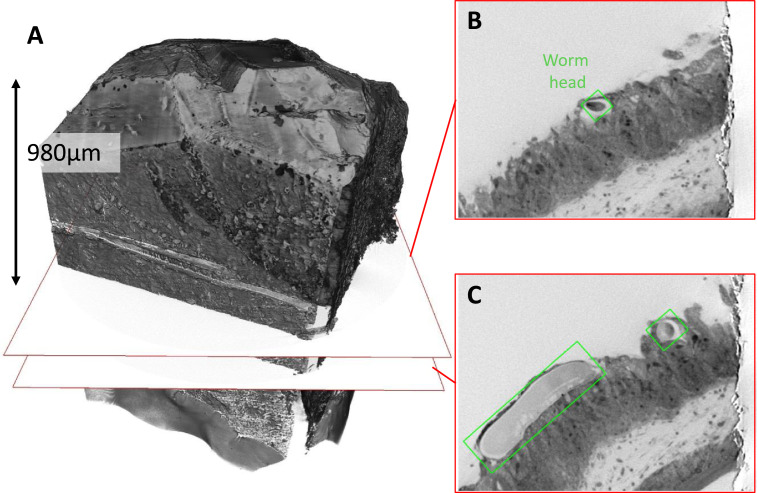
Identifying regions of interest within the X-ray data set to target subsequent trimming.*(A) A magnified volume render of the resin sample generated from the X-ray data. (B) Virtual XY section showing the worm head buried within the cecum tissue. Analysis of the X-ray tomogram shows that this feature is found 980 μm below the current block-face. (C) A virtual XY CT slice showing the same worm head 180 μm deeper into the sample. At this point it is also possible to see part of the body of the whipworm (within the green rectangle, modelled in 3dmod).
